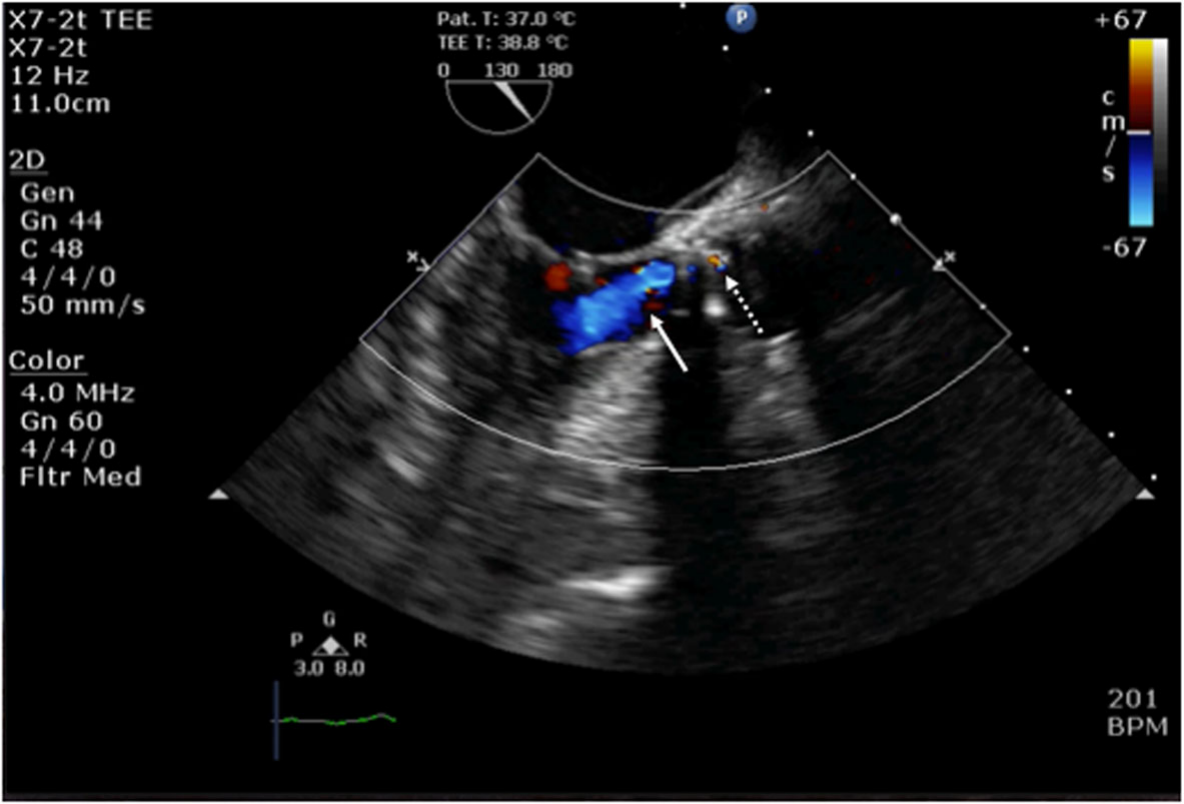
A mid-esophageal aortic valve long-axis transesophageal echocardiography image reveals an aortic regurgitation jet and its acceleration flow. White arrow indicates aortic regurgitation, and white dotted arrow indicates its acceleration flow
